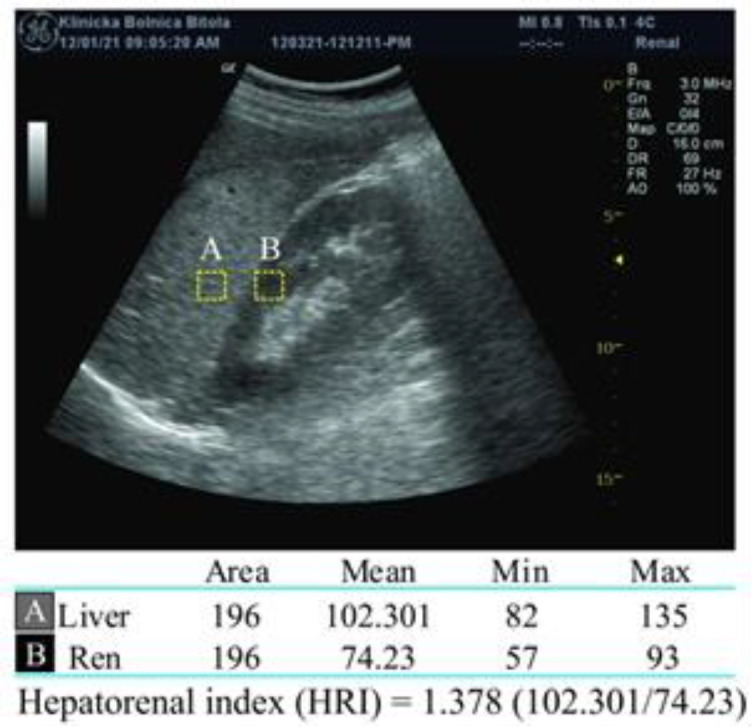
Analysis of an ultrasound image using ImageJ software and calculation of the hepatorenal index
